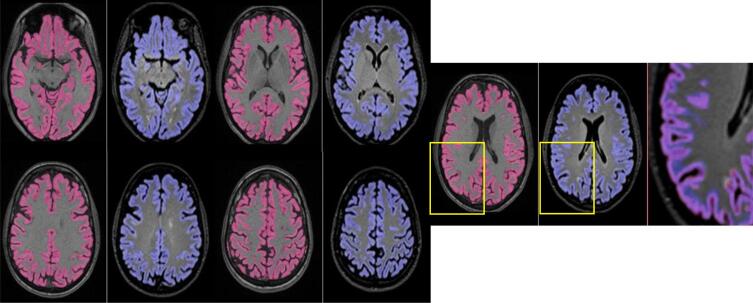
A subject’s cortical GM segmentation shown for 1.5T, using the multimodal GIF database. T1 segmentation is denoted in pink, and T2-FLAIR segmentation is shown in blue. An enlarged image overlaying both T2-FLAIR and T1 segmentations is included on the right of each series, showing areas of discrepancy, highlighted in the yellow boxes.
